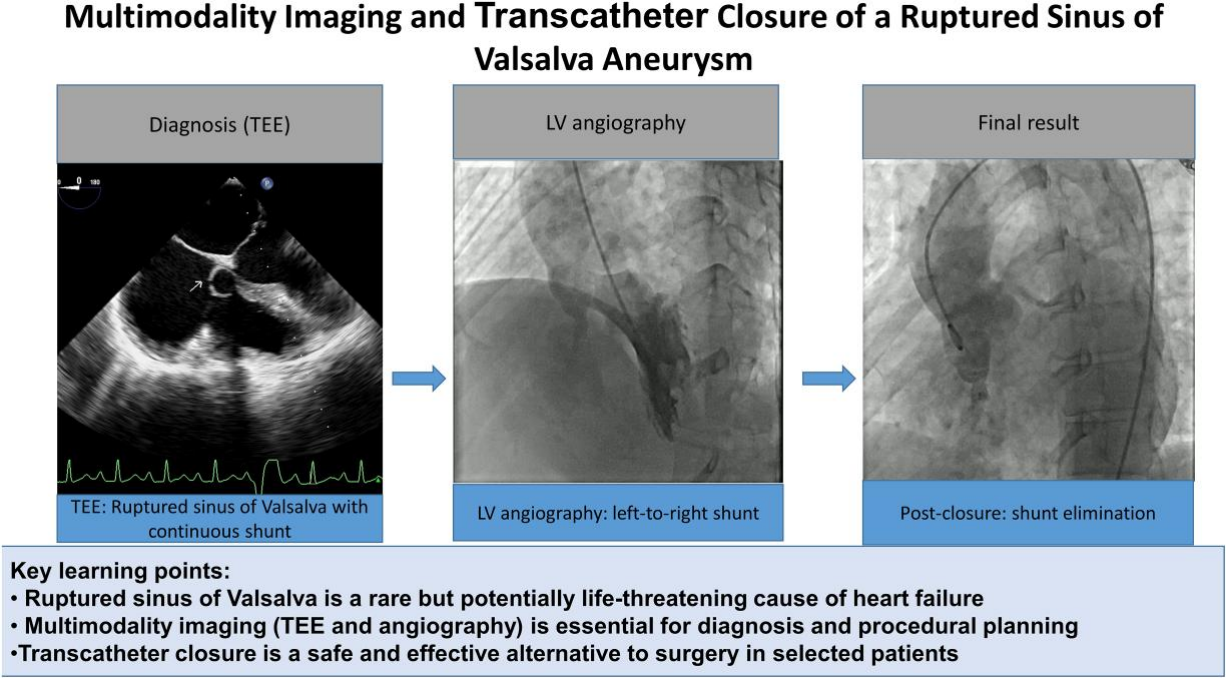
Multimodality imaging and percutaneous management of a ruptured sinus of Valsalva aneurysm. Transoesophageal echocardiography confirms rupture with left-to-right shunt into the right ventricle. Angiography demonstrates the fistulous communication. Final fluoroscopic imaging shows successful transcatheter device closure with elimination of the shunt.
